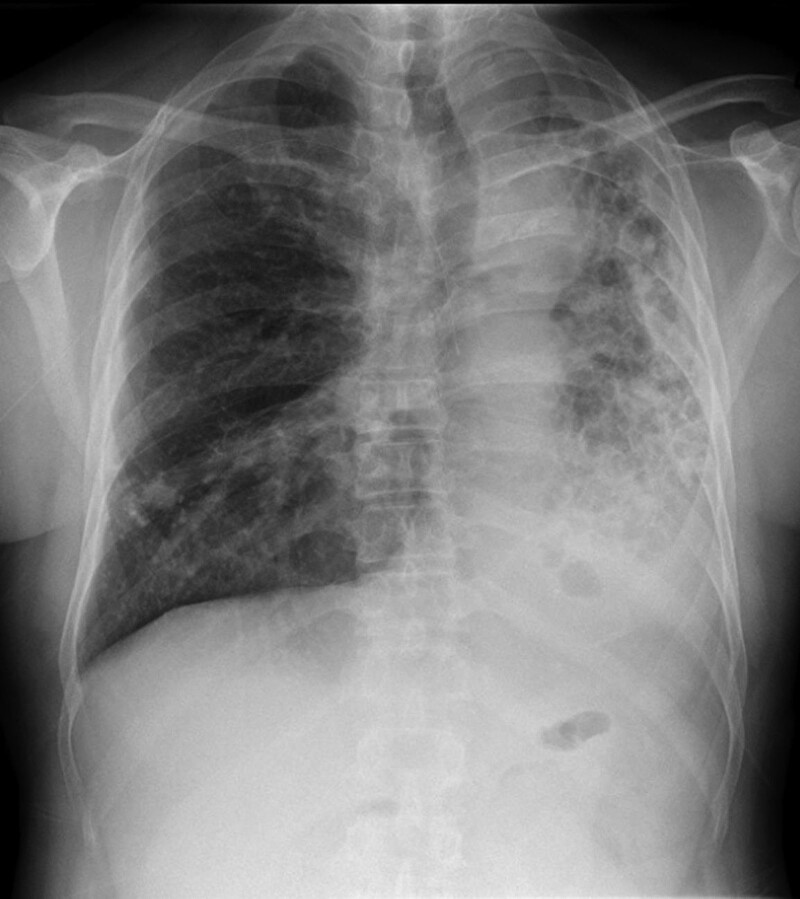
Chest radiograph image showing a decreased volume of the left lung, which was significantly smaller than the right lung.
